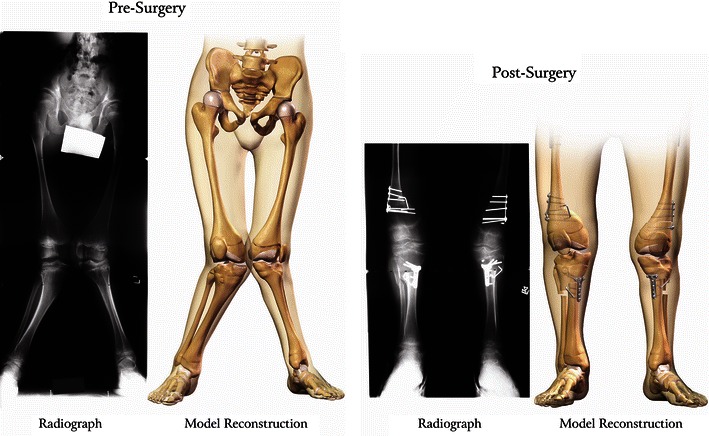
Representative radiographs and computer models of the pathoanatomy pre- and post-reconstruction for a single patient (patient REF # 10). The pre-reconstruction radiographs were taken when the patient was 16 years of age; post-reconstruction 2 months after surgery. The angle between the femoral shaft and tibial mechanical axis was 28° right and 29° left pre-reconstruction, and 0° right and 7° left post-reconstruction
